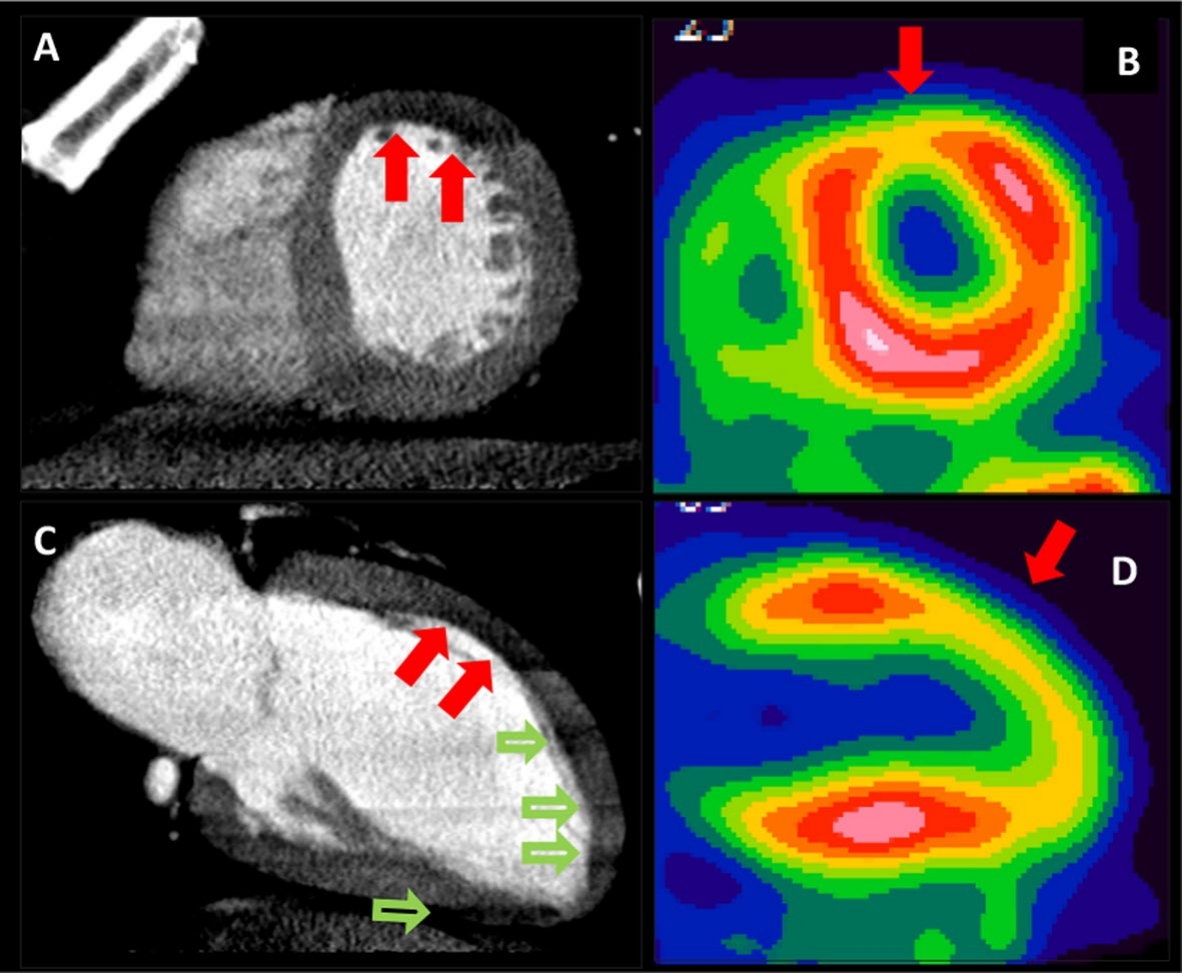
Stress CT-MI and ^82^Rb-PET agreement by visual analysis. Post dipyridamole stress short-axis images of CT-MPI in (A, C) and ^82^Rb-PET (B, D). Perfusion defects on post dipyridamole stress CT-MPI in mid anterior segments and corresponding ^82^Rb-PET images in a single patient (red arrows). Note beam hardening artifacts in apical segments in CT images (open green arrows)
